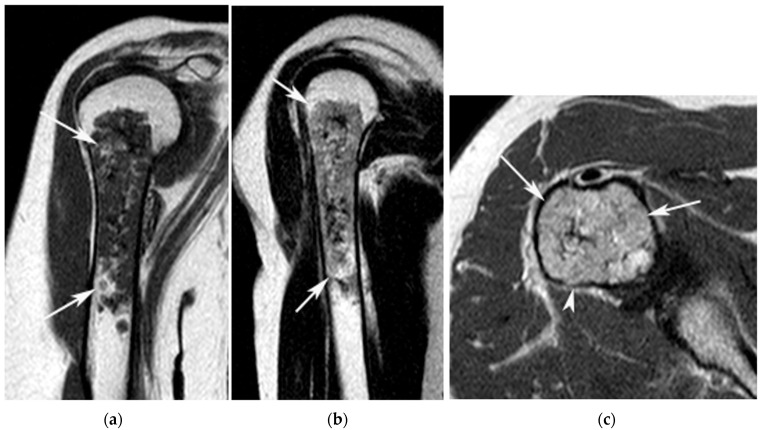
A 58-year-old female with an ACT in the right proximal humerus. (a) Coronal T1W TSE, (b) sagittal T2W FSE and (c) axial PDW FSE MR images show an extensive chondral tumour (arrows) centred on the proximal metaphyseal region of the humerus. The lesion fills the medullary cavity but does not result in any bone expansion or cortical destruction.
